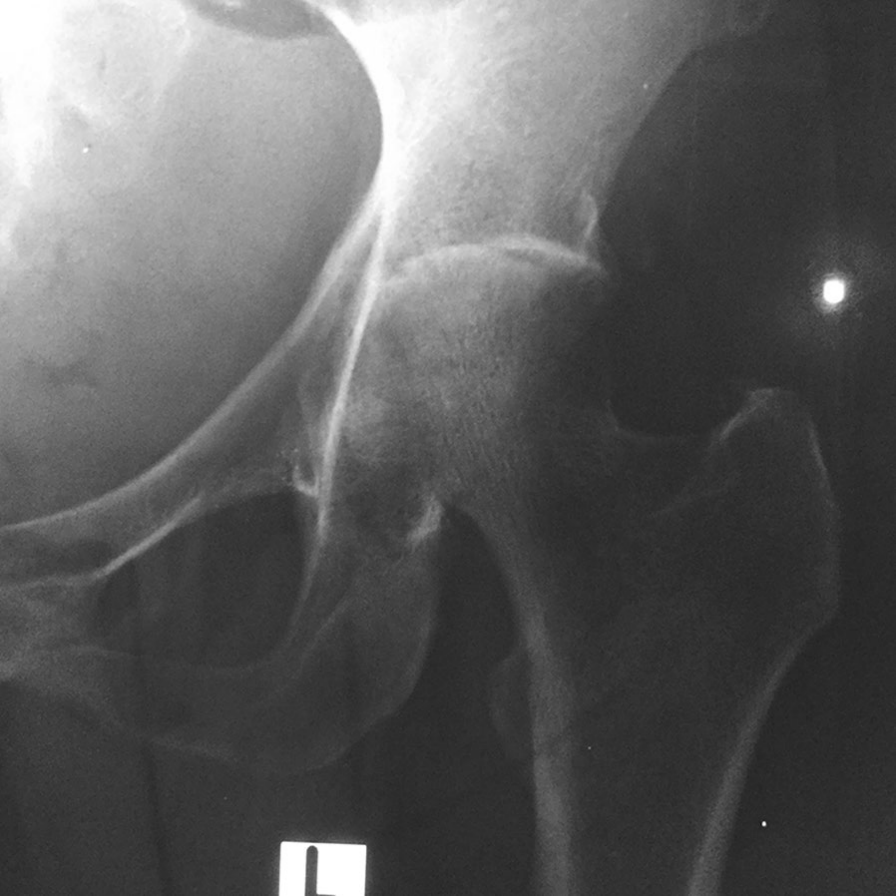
X-ray of the left hip showing narrow joint space and destruction of femoral head
